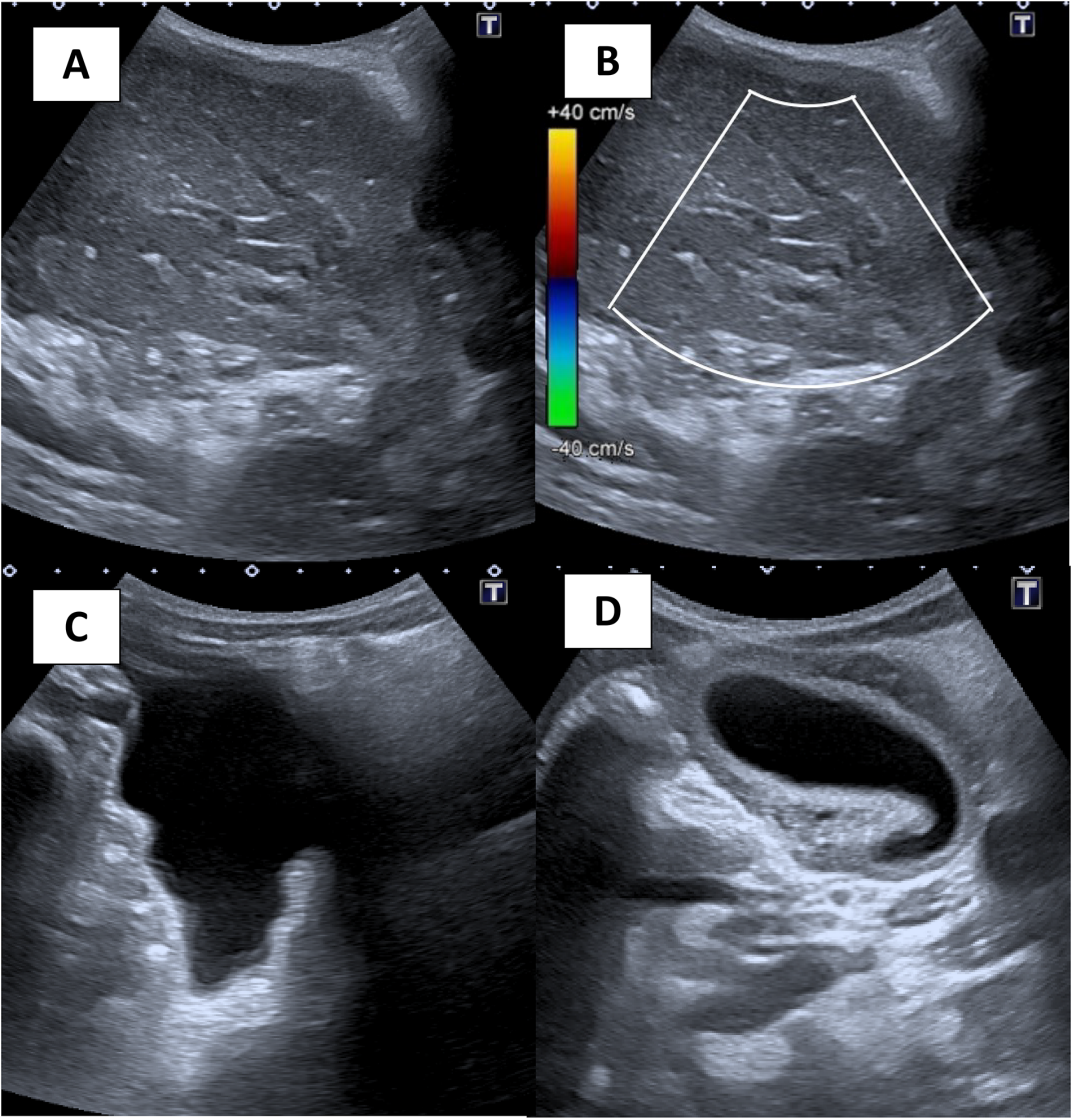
(A) grayscale ultrasound image of the right abdomen shows a mass that is mostly hypoechoic and heterogeneous. (B) Color flow Doppler image demonstrates no color flow within the mass. (C) Free fluid in the pelvis. (D) A gallbladder that is displaced to the left and with a thick wall.
